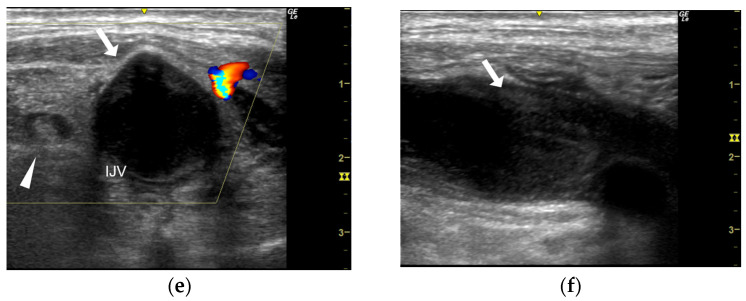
Various thrombus shapes (arrows). (a,b) Fibrin sheath (FS) is a thrombus that appears as a cord-like structure. (c,d) Images show FS with mural thrombus. (e,f) Images show thrombus filling and occluding the IJV. Color Doppler images show no blood flow in the IJV. An enlarged lymph node (arrowhead) near the obstructed IJV was also observed. IJV, internal jugular vein; CCA, common carotid artery.
